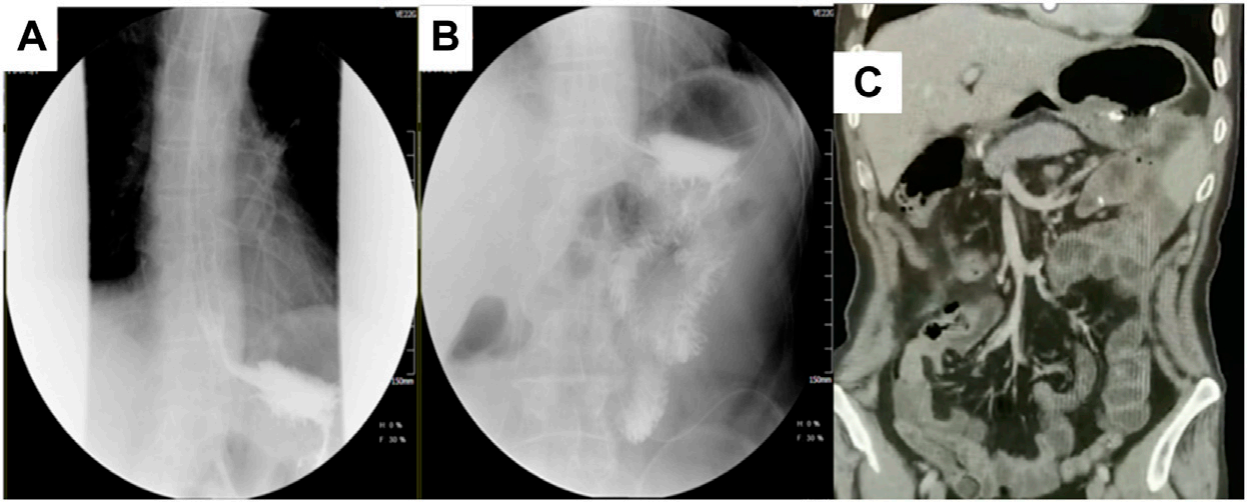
Postoperative upper gastrointestinal angiography and CT coronal plane. (A,B) showed that the reconstructed digestive tract is unobstructed, and the anastomosis is normal. (C) showed no obvious signs of recurrence and metastasis after surgery and another three cycles of adjuvant treatment.
